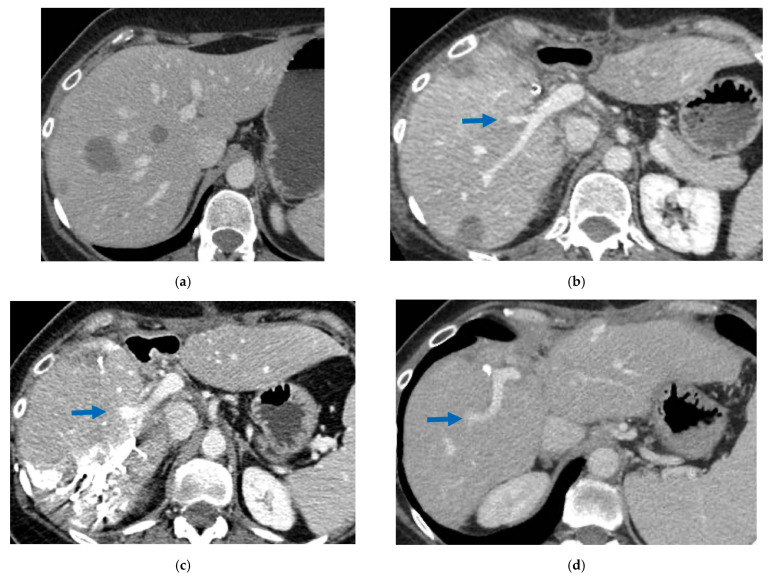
CT Images of PS-TSH with SS-PVE performed to treat 35 bilateral CRLMs. (a) Partial response after FOLFOXIRI + Bevacizumab 12 cycles; FLR (=S2/S3) volume is 15%. (b) Blue arrow points GP 5 after the first stage consisting of resection of S1/S4 extended to S2/S8/S5 plus metastasectomy of S2 with tangential resection of LHV. (c) Fourteen days after stage 1 SS-PVE was performed on the right liver preserving GP 5 (blue arrow); 37 days after SS-PVE FLR (=S2/S3/S5) increased up to 37%. (d) Blue arrow points GP 5. Twenty-five months after PS-TSH with SS-PVE the patient is disease free; the liver’s only disease recurrence was successfully treated with another 3 liver resections (2 on the right liver and 1 on the left). Abbreviations: PS-TSH: parenchyma-sparing TWO-STAGE hepatectomy; SS-PVE: super-selective portal vein embolization; CRLM: colorectal liver metastases; FLR: future liver remnant; S: segment; GP5: glissonean pedicle for segment 5; LHV: left hepatic vein.
